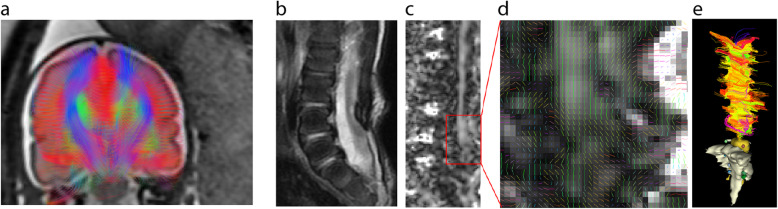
Diffusion tensor imaging in spinal dysraphism. a Diffusion tractography of a foetus with spinal dysraphism, 26th week of gestation. The fibre tracts (colour representing spatial orientation) have been overlaid on a coronal plane, T2-weighted MR image. b Sagittal T2-weighted MRI of a newborn with spina bifida, corrected prenatally, showing signs of tethered cord. c Sagittal fractional anisotropy image of the same myelon cross-section, demonstrating increased anisotropy in the region of the tethering. d Diffusion tensor principal vector orientations in the same region. e Diffusion tractography of the myelon
